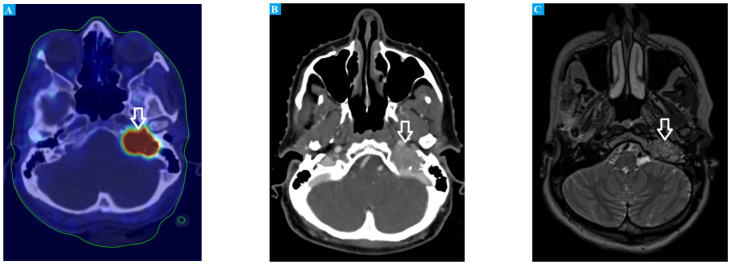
(A) combined ^68^GA-DOTATE PET and CT images showing a large, highly metabolically active mass in the skull; (B) CT scan showing a polycystic tumor compressing the left cerebellar tonsil; (C) combined MR and CT scan showing infiltration of the left sublingual nerve extending to the left internal carotid artery canal and obstructing the left internal jugular vein at the inferior bulb region.
